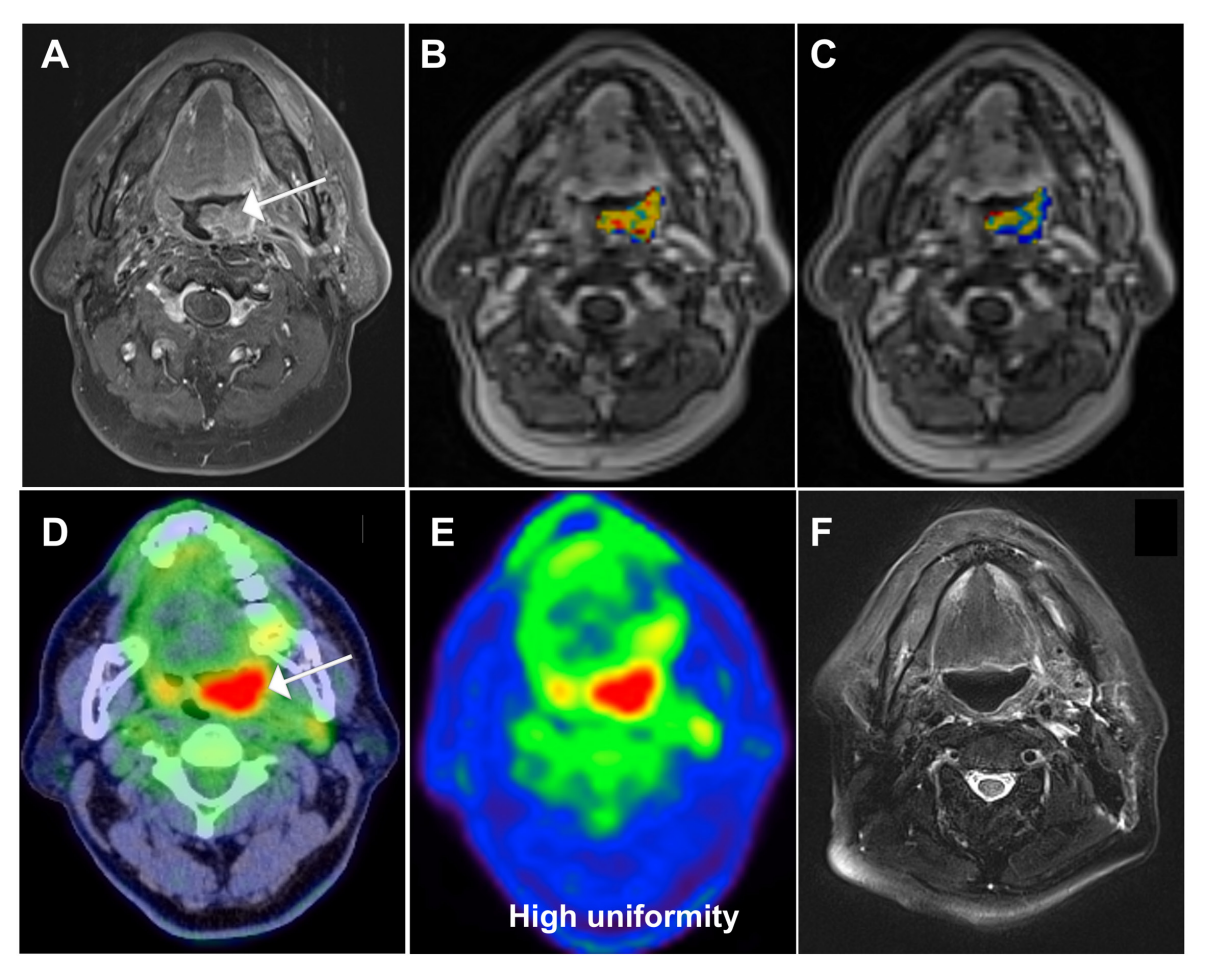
A 62-year-old male patient with oropharyngeal squamous cell carcinoma and a score of 1 based on the prognostic system developed in the current study A. Pretreatment axial-enhanced MRI image showing a left oropharyngeal tumor (arrow). B. The corresponding DCE-MRI image with an overlaid K^*trans*^ map of the primary tumor showed a value of 1.056 min^−1^. C. The corresponding DCE-MRI image with an overlaid K_*ep*_ map of the primary tumor showed a value of 1.1356 min^−1^. D., E. The corresponding ^18^F-FDG PET/CT image showed a uniformity value of 0.0047 and a SUV of 15.61 g/mL. F. Post-treatment axial-enhanced MRI showed complete regression of the primary tumor. After 41 months of follow-up, the patients remained disease-free. Of note, this case would have been classified as having a poorer outcome (with a score of 2) using the modified prognostic scoring system reported in the previous study (Table 3).
